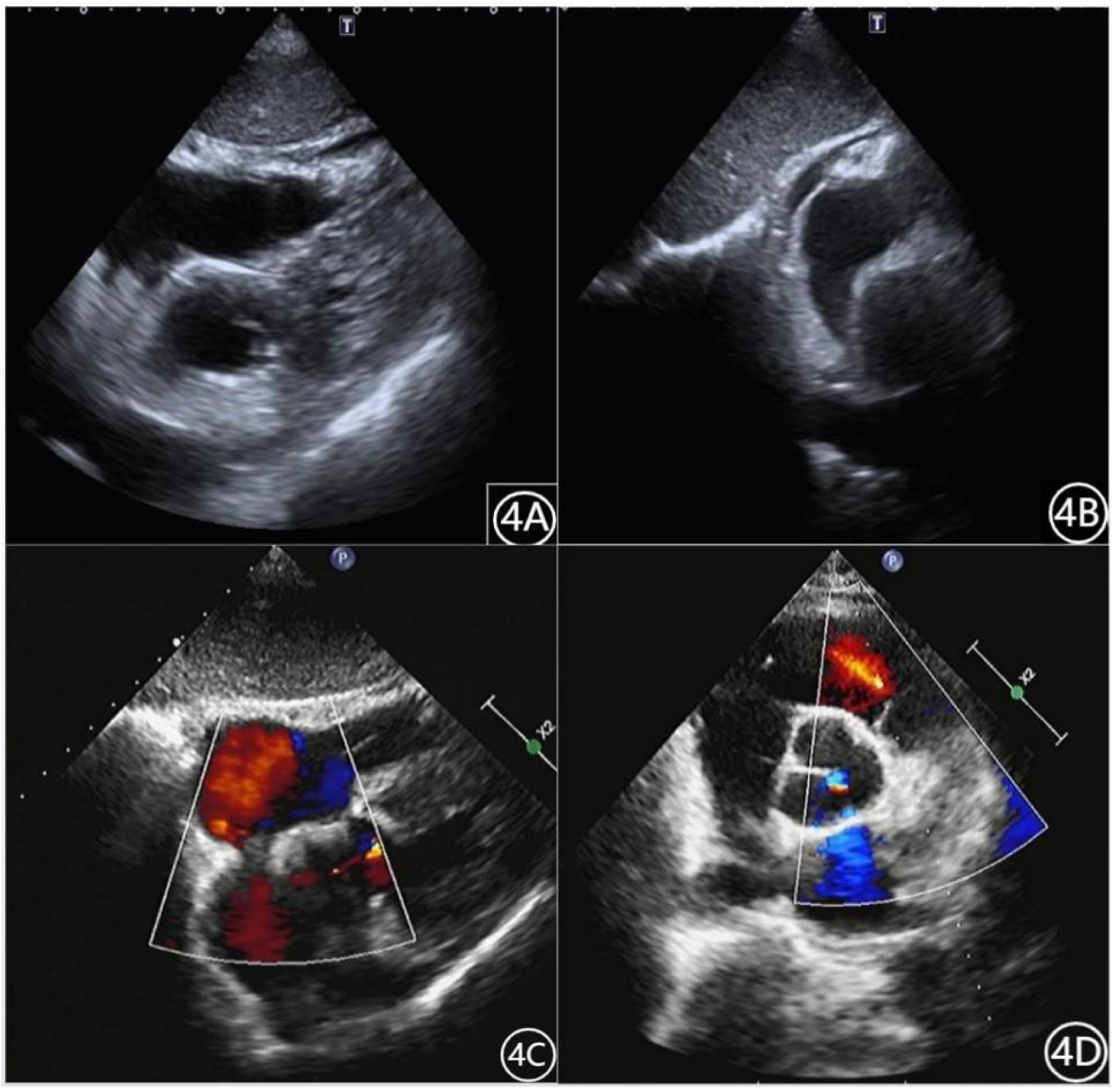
Patient's cardiac ultrasound data (A): May 23, 2022. (B): May 28, 2022. (C): June 6, 2022. (D): July 8, 2022.
